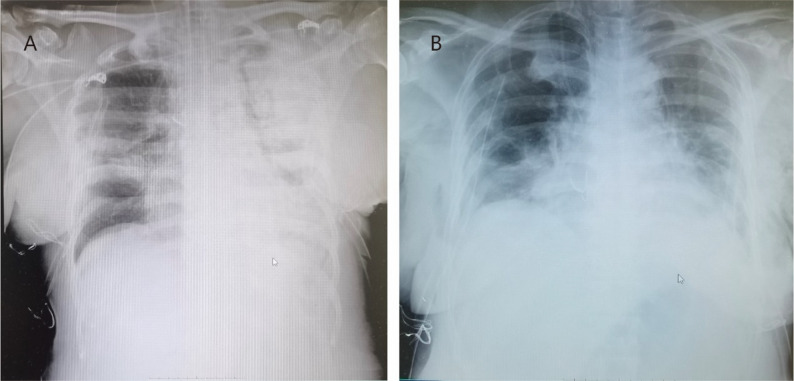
Chest radiograph obtained on the first day(A) and fifth day(B) after surgery
